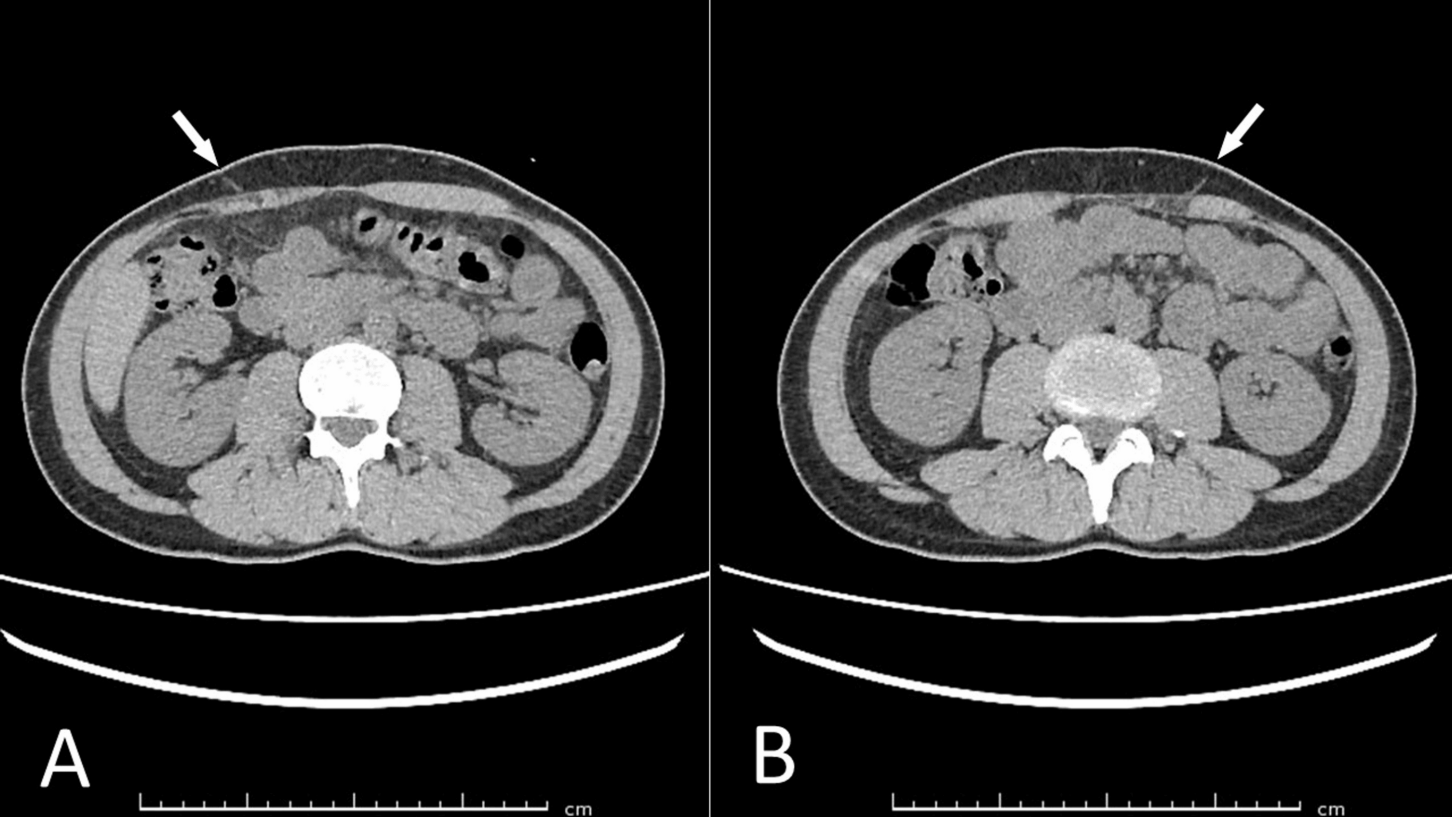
Preoperative CT images of the abdominal wall in case five Axial CT image of the abdominal wall. (A) The white arrow indicates a visible anterior cutaneous nerve bundle. Note the localized thinning of the right rectus abdominis and thickening of the anterior sheath fascia, consistent with chronic mechanical compression. (B) The white arrow points to a similarly visible cutaneous nerve bundle. Although the patient reported no pain on this side, focal atrophy of the rectus abdominis suggests subclinical muscle degeneration and potential nerve involvement.
